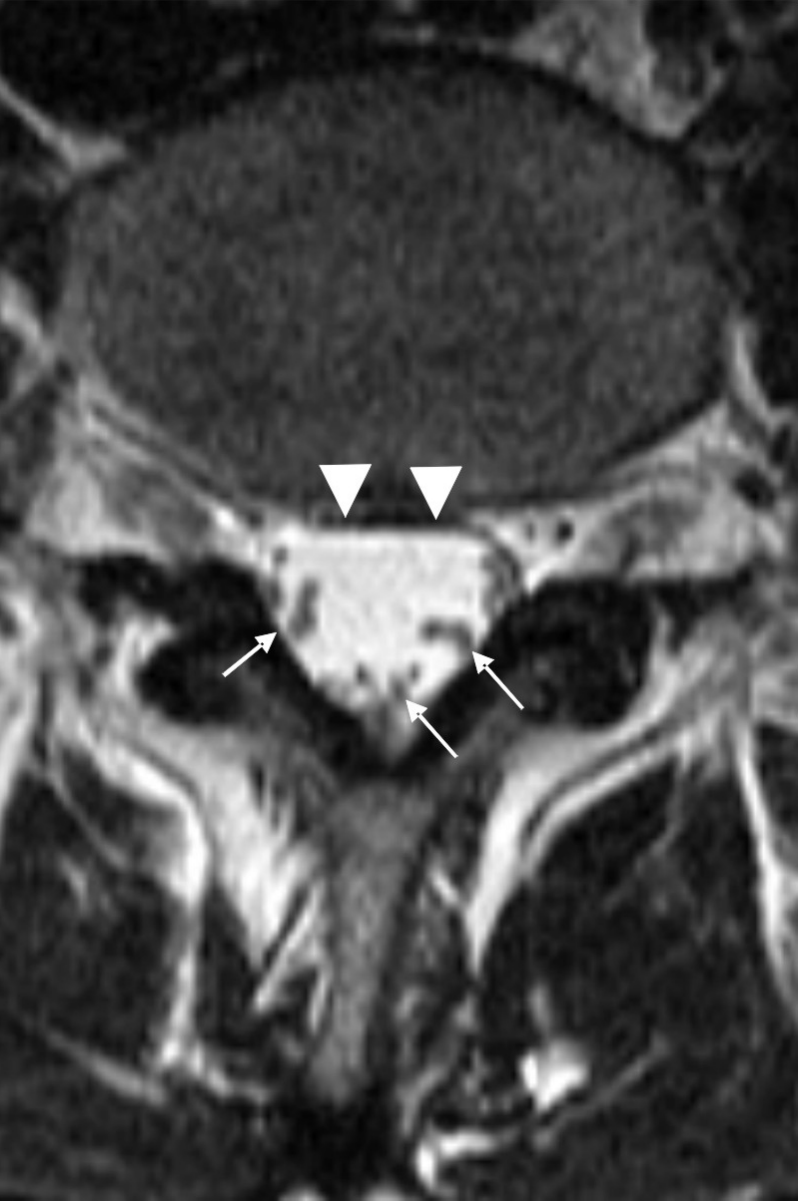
Magnetic resonance images of a normal lumbar spine. Cross-sectional magnetic resonance images of a normal lumbar spine with dural sac cross-sectional area of 180 mm^2 ^and LEL 0 (according to Borré). On the T2-weighted image, the cerebrospinal fluid appears nearly white and the nerve roots are more easily seen in the large cerebrospinal volume than on a T1-weighted image. The nerve roots are free to move away in the cerebrospinal fluid space when a needle is inserted into the dural sac.
